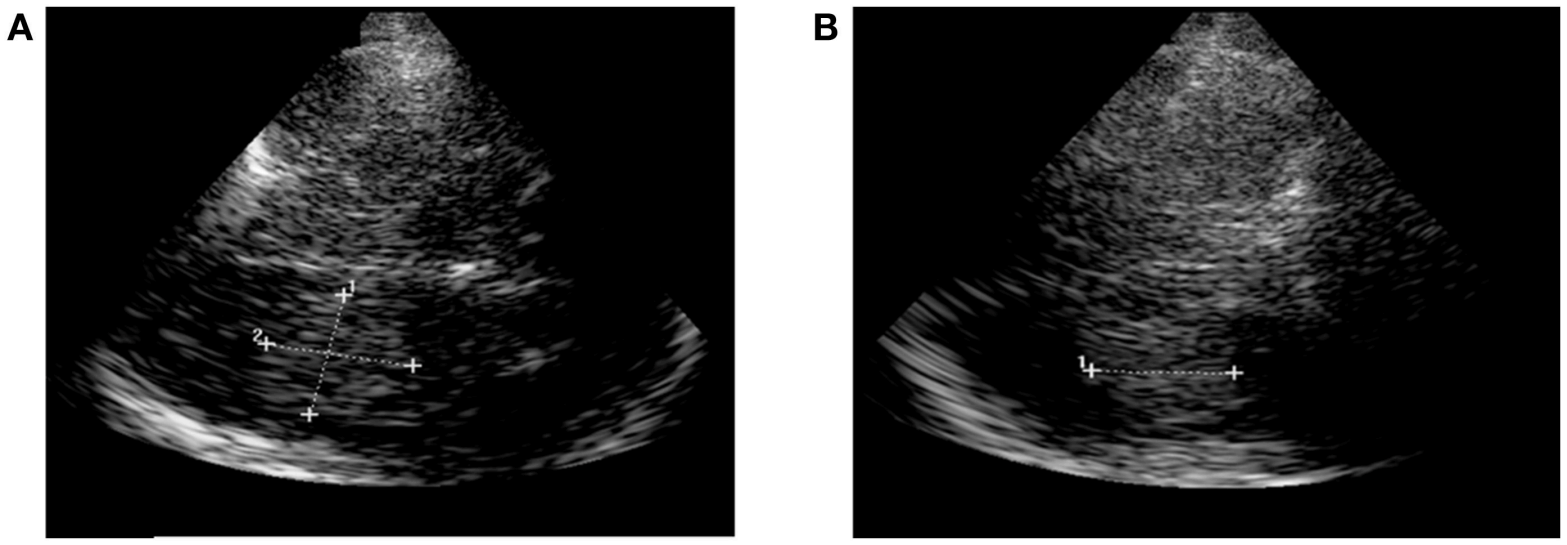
Sonographic ICH-volumetry. (A) Hemorrhage expansion measured in the axial plane (length and width of the ICH). (B) Hemorhage expansion measured in the coronal plane (height of the ICH). ICH, intracerebral hemorrhage.
